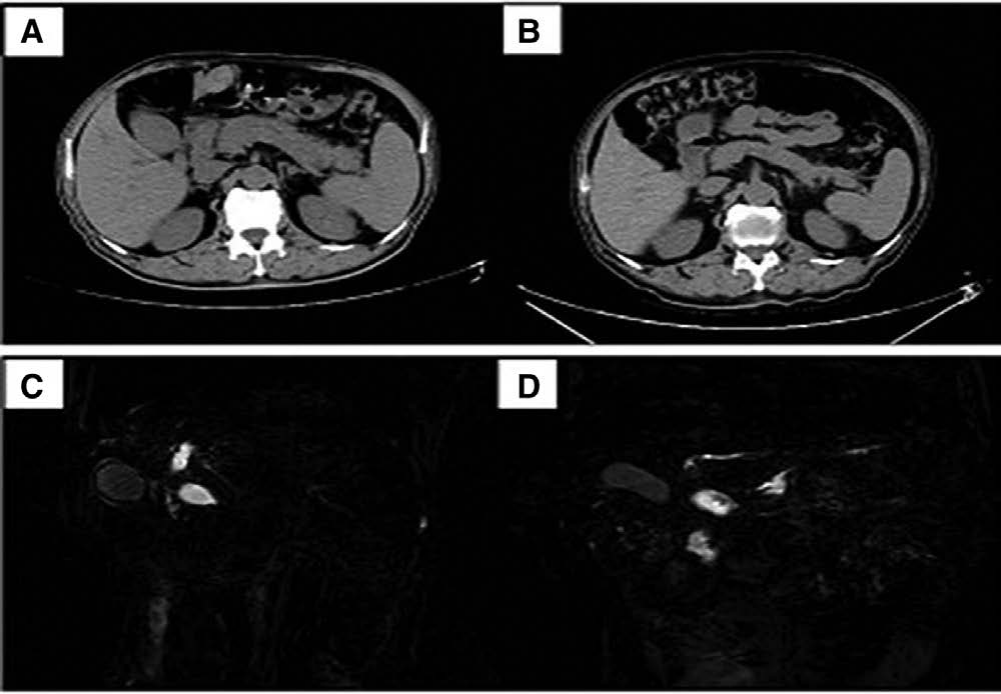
Serial images from a 67-years old man with positive steroid responsiveness who was finally diagnosed as type-1 autoimmune pancreatitis. (A, C) Pretreatment: CT showed diffuse enlargement of the pancreas, and endoscopic MRCP showed narrowing of the main pancreatic duct. (B, D) Post-treatment: after the 2-weeks steroid trial, the pancreas was reduced in size and the main pancreatic duct stenosis was significantly improved. CT = computed tomography, MRCP = magnetic resonance cholangiopancreatography.
